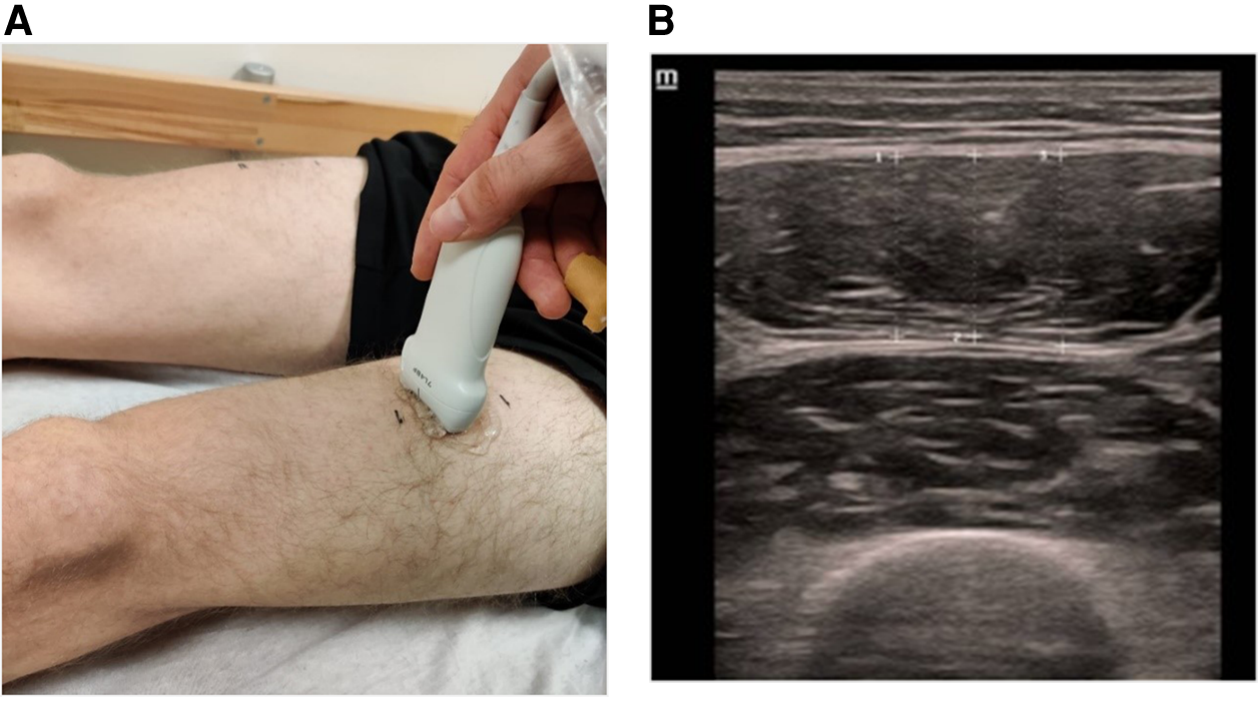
Sonography to measure muscle thickness of m. rectus femoris (A) and image analysing of three subsequent distances (B).
